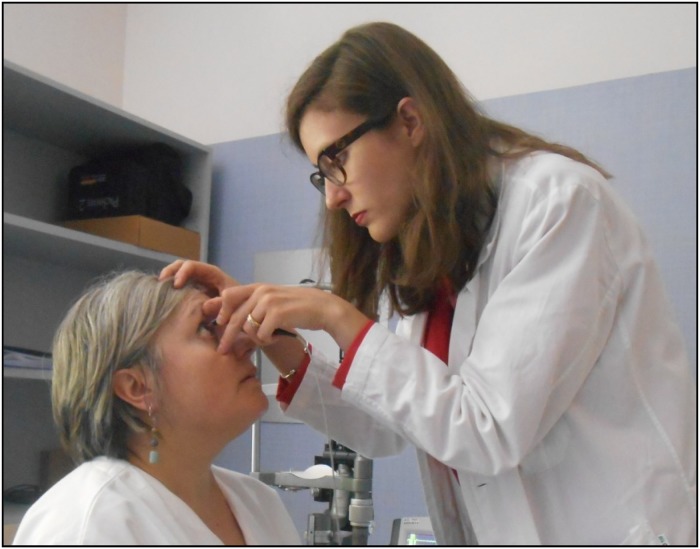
The execution of pachymetry: the ultrasound probe shall be placed on the corneal apex. Written informed consent was obtained from the individuals for the publication of this image.
